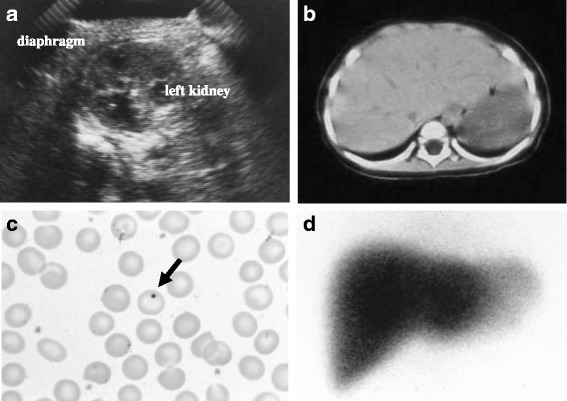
a Ultrasonography of the abdomen indicating the absence of a spleen; the upper pole of the left kidney is located beneath the diaphragm. b Nonenhanced computed tomography of the abdomen indicating the lack of a spleen on the left. c Peripheral blood smear showing the presence of Howell-Jolly body (arrow). d Scintigraphy with Tc-99 m phytate colloid showing no functional spleen tissue
